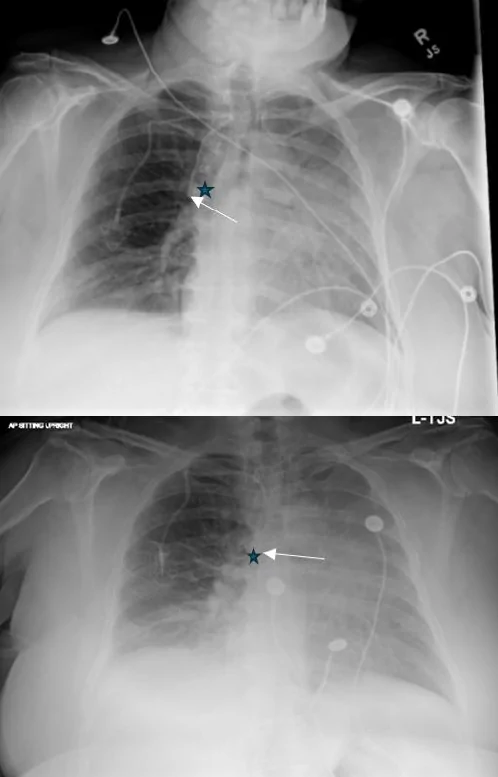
Top: Anteroposterior (AP) view chest radiograph immediately after central venous catheter placement, demonstrating radiologic confirmation of appropriate placement with the tip overlying the expected location of the lower superior vena cava. Bottom: AP view chest radiograph upon presentation to the emergency department. Arrow is pointing to the tip of the central venous catheter. Star indicates the location of the superior vena cava, which is located to the right side of the mediastinum above and below the level of the carina.
